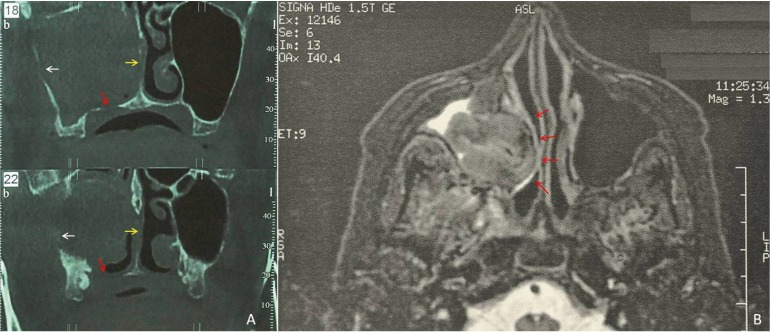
(a) Coronal Computer Tomography showed a solid, voluminous, hypointense lesion occupying the middle and inferior turbinate and the whole right sinus cavity, with thickening of the sinus mucosa and perforation of the sinus floor (red arrow) and the lateral nasal wall (yellow arrow). (b) A prominent expansion of the lateral nasal wall was observed on the magnetic resonance imaging (red arrows).
